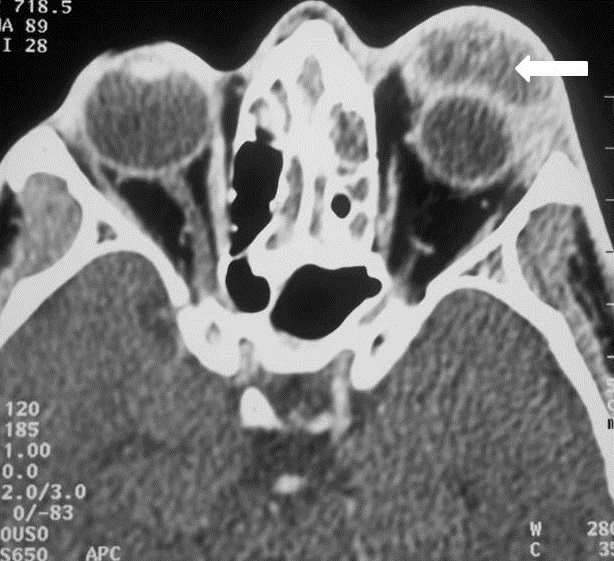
CT of the facial mass (from the same patient in figure 1) in axial sections after PDC injection in the soft-tissue window showing a presptal hypodense orbital image with peripheral contrast check (arrow): left prespetal abscess (Chandler’s Stage I) complicating frontal homolateral sinusitis
